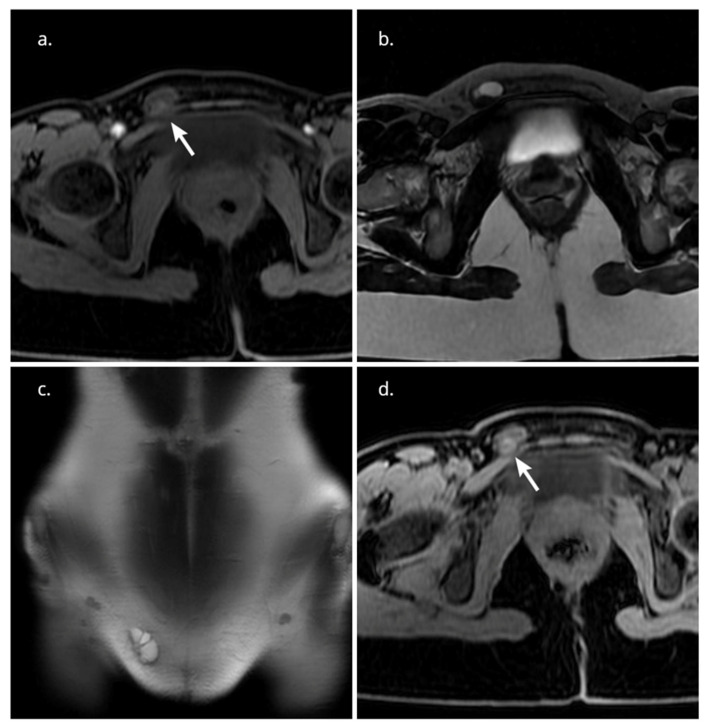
(a) Axial T1-weighted images with fat suppression: 2.8 cm hypointense lesion in the right inguinal canal with small hyperintense, probably hemorrhagic foci in the posterior part (white arrow). (b) Axial T2-weighted images: the lesion demonstrates hyperintense signal with hypointense foci in its posterior part. (c) Coronal T2-weighted images: hyperintense lobulated, septated cystic mass in the right inguinal canal. (d) Axial T1-weighted images with fat suppression after administration of gadolinium-based contrast material: Slightly enhancing foci in the posterior part of the lesion (white arrow).
